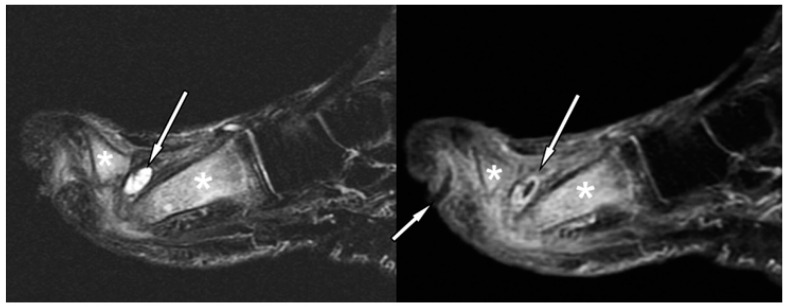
Forefoot ulcer, sinus track, and abscess associated with OM in a 57-year-old diabetic man with a 16-year history of insulin-dependent diabetes. Sagittal T2 fat-suppressed (left panel), and post-contrast T1-weighted fat-suppressed MRI (right panel) shows a dorsal thick rim-enhancing abscess adjacent to the first metatarsal head (arrows). Note a plantar ulcer appearing as a focal skin interruption and a sinus tract with rim-like enhancement (small arrow in right panel) extending near the first proximal interphalangeal joint. Given these findings, the hyperintensity ( in left panel), and post-contrast enhancement ( in right panel) in the first metatarsal, and proximal phalanx respectively, are indicative of OM.
